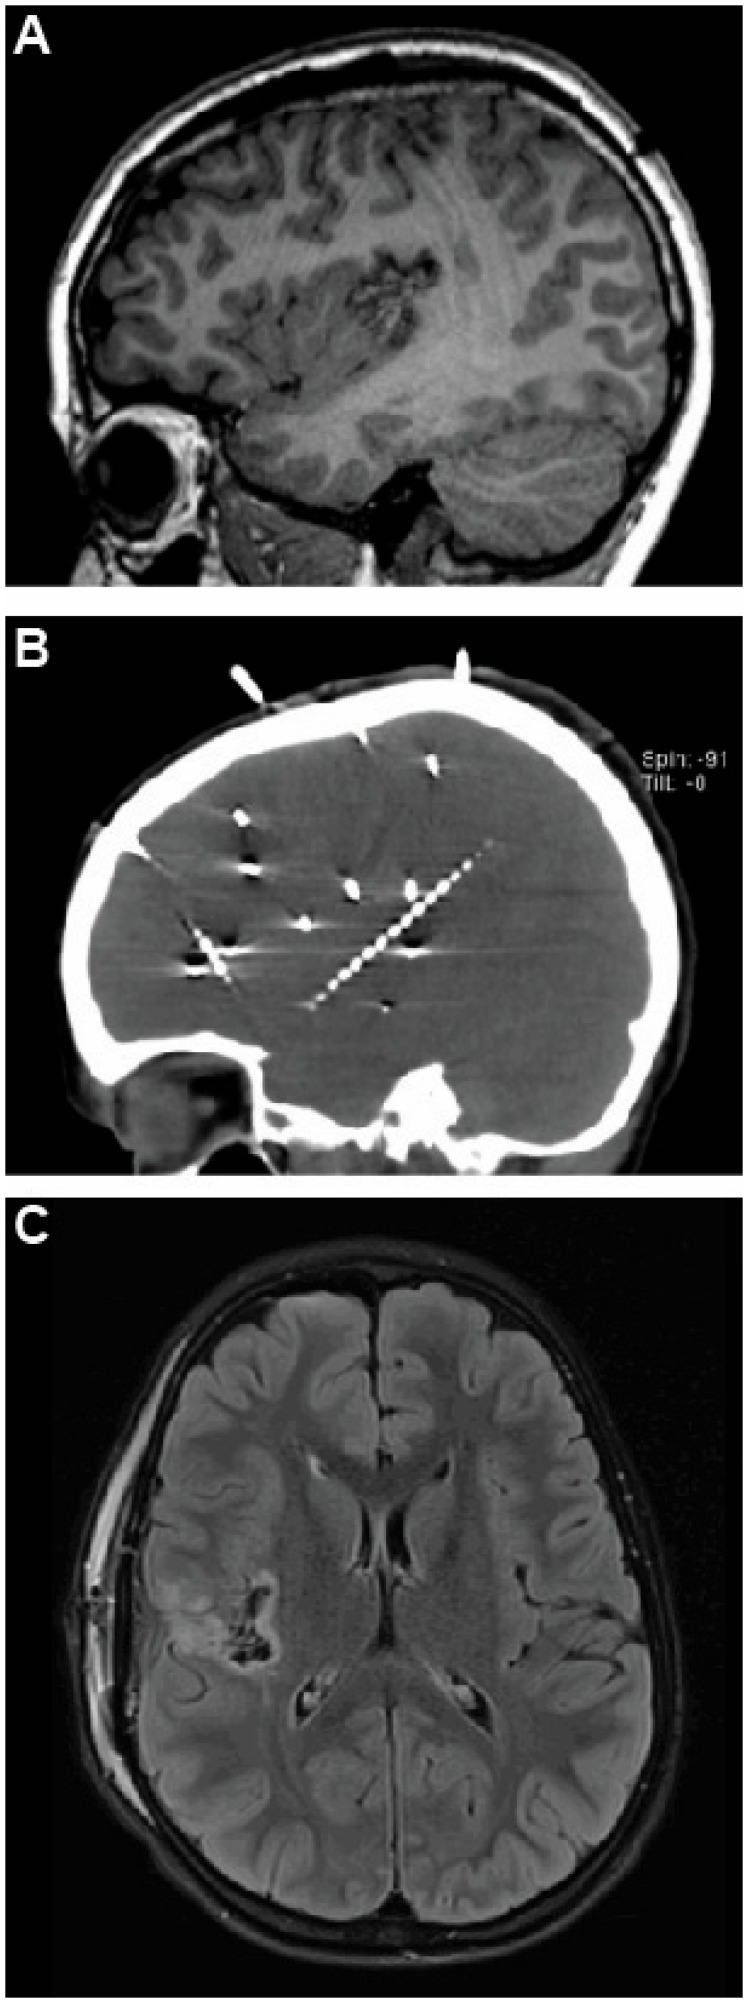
Postoperative sagittal T1-weighted MRI for Case 1 showing resection cavity (A), with prior sEEG CT scan for comparison (B). Postoperative FLAIR MRI showing posterior insular resection without corona radiata infarct (C).
